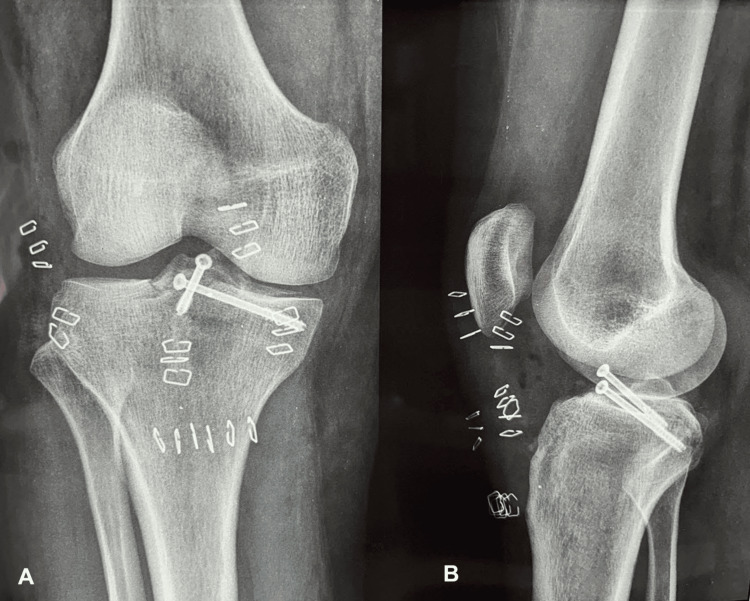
Knee radiographs just after the operation where it can be noticed that the anatomic reduction has been achieved. (A) Anteroposterior view. (B) Lateral view: internal fixation with two antegrade cannulated screws.
